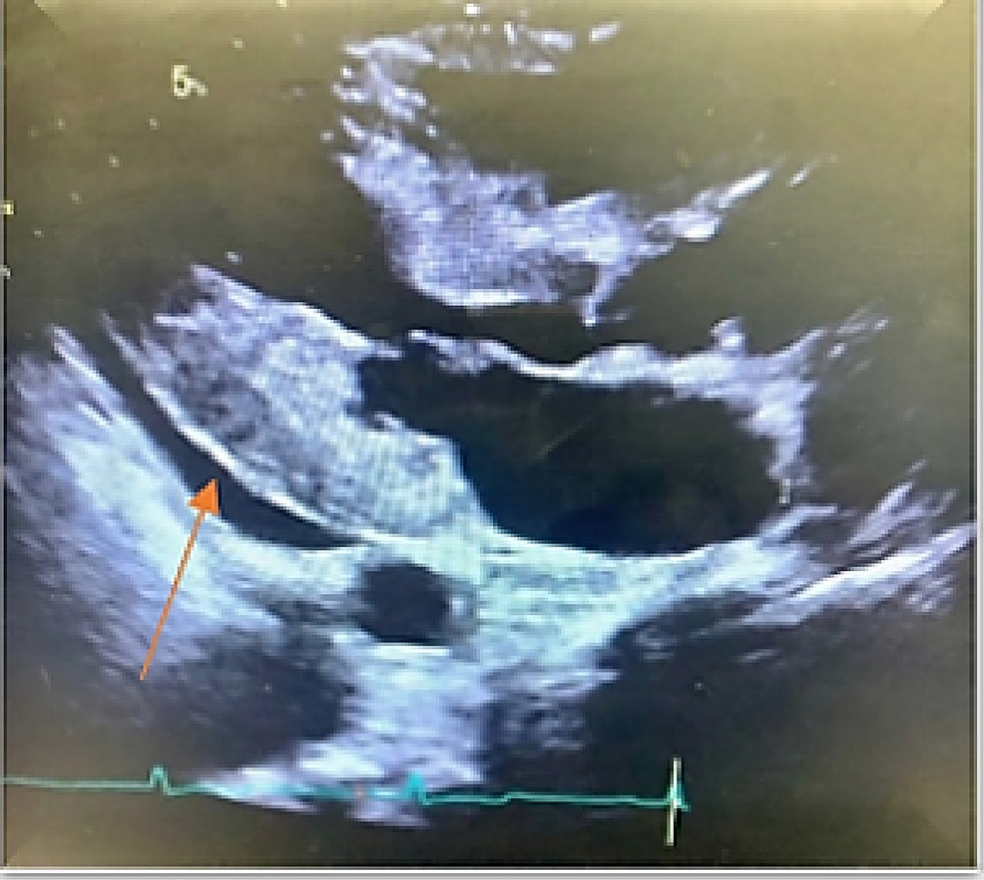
Two-dimensional echocardiography long-axis view showing pericardial effusion.
